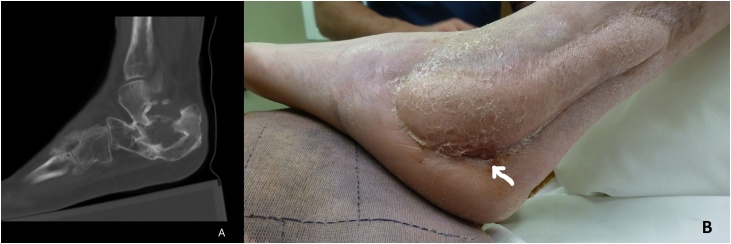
A – Calcaneal osteomyelitis in MRI. B – Pre-operative photograph with sinus tract (arrow).
